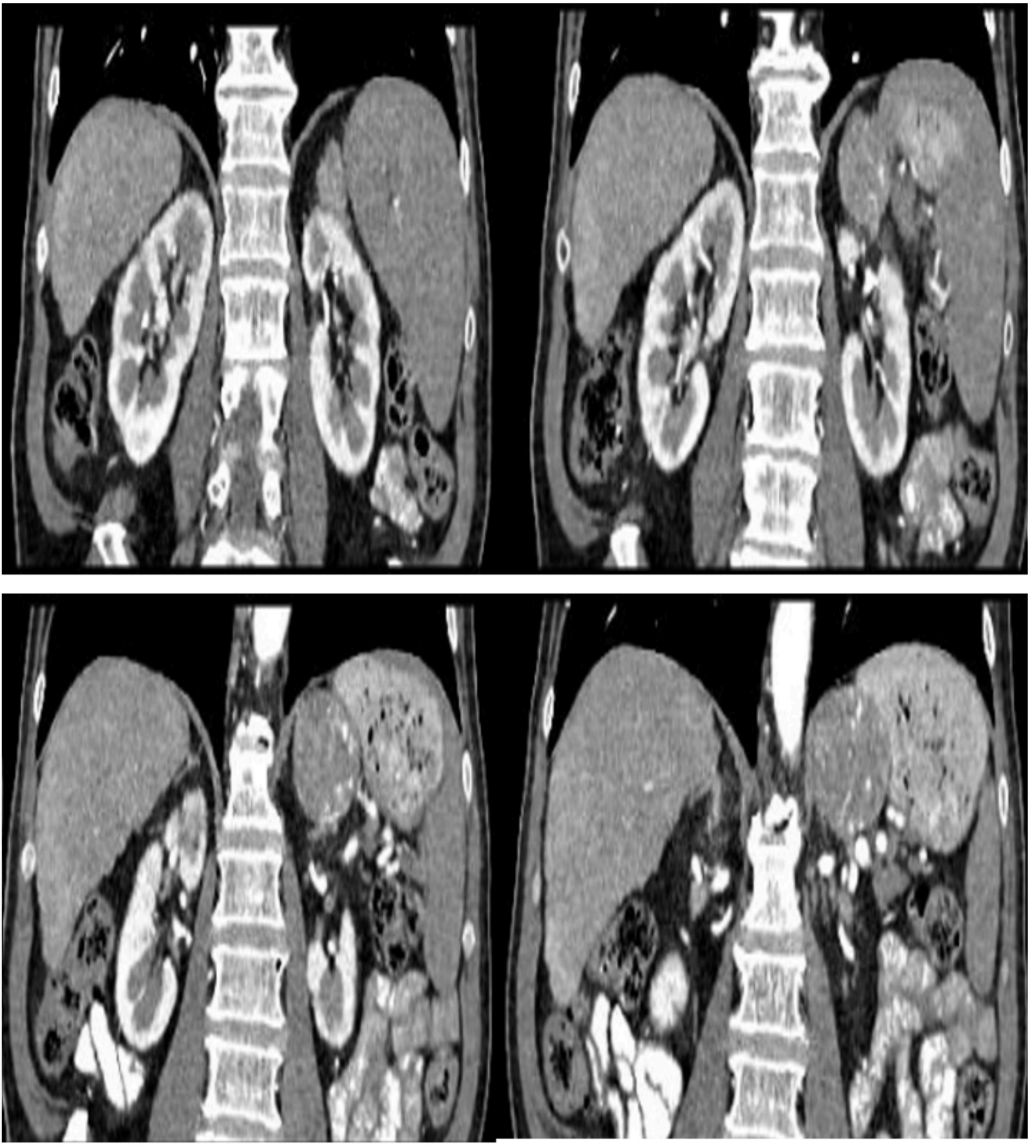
Contrast abdominal-pelvic computed tomography. Left adrenal gland with solid lesion with heterogeneous contrast uptake, with central and peripheral penetrating vessels, size approximately 4.8 cm in diameter. No fatty content or calcifications were observed in its interior.
